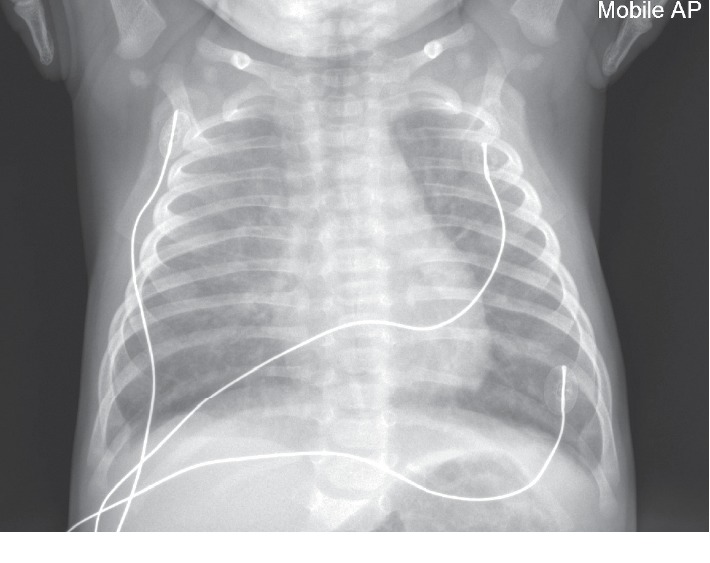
Chest X-ray of infant on presentation with diffuse bilateral ground glass changes.
